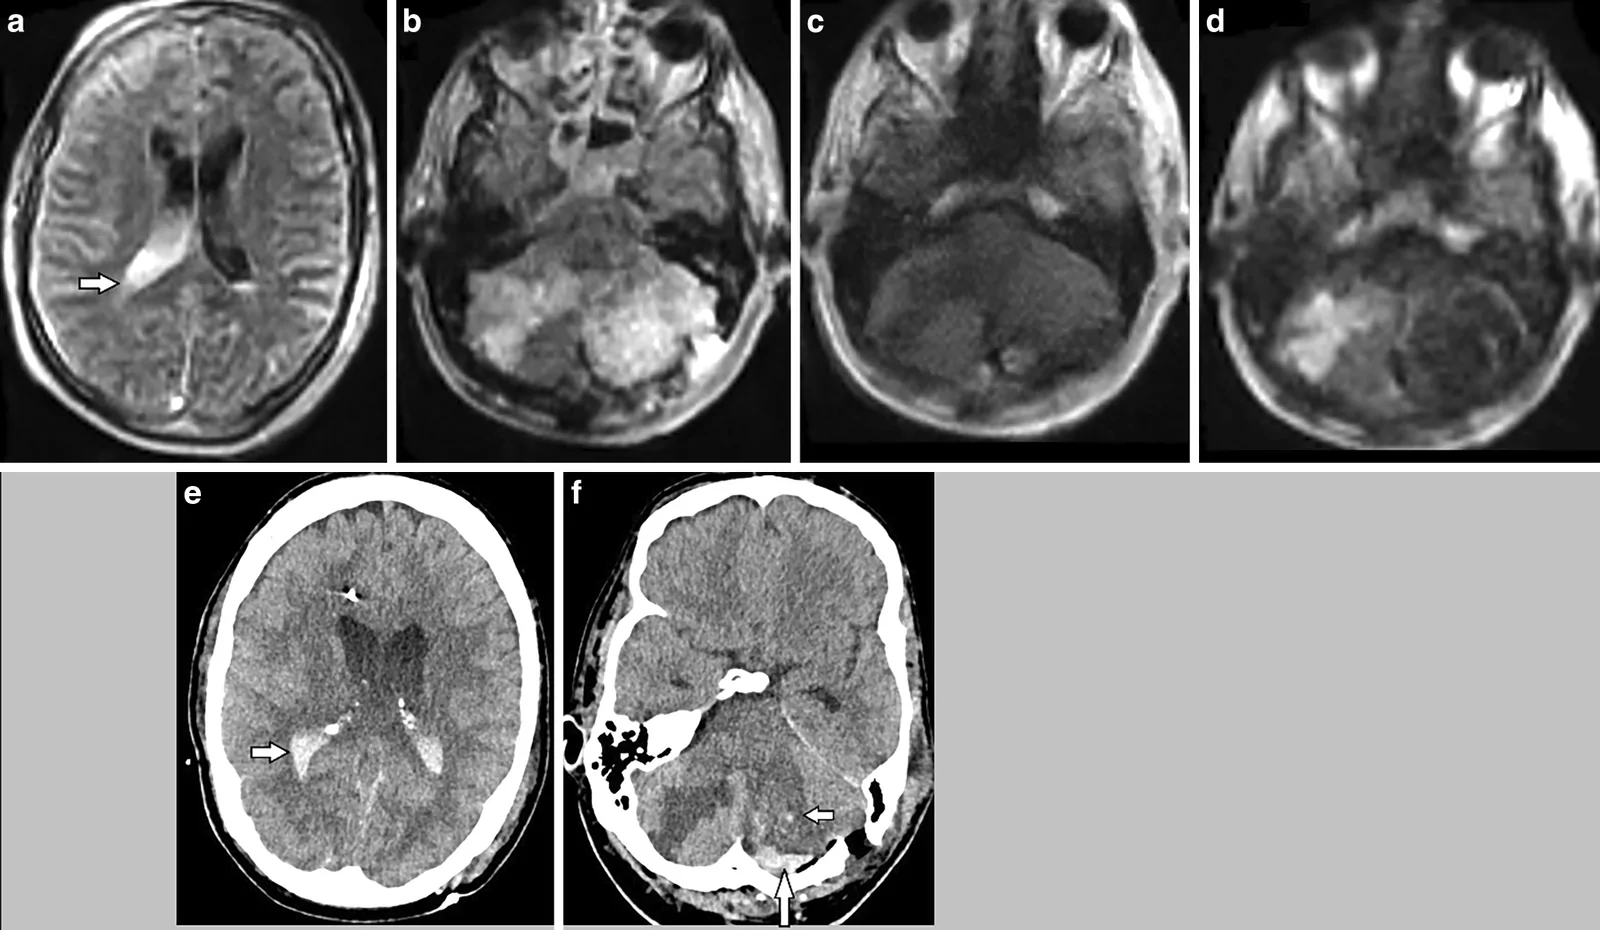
a 57-year-old patient with bilateral cerebellar infarctions with hemorrhagic transformation. ULF-pMRI showed massive intraventricular hemorrhage (HBC3b), predominantly in the right lateral ventricle (arrow in a FLAIR ULF-pMRI; e CT). Hemorrhagic components were clearly visible only on CT, which was obtained after surgical decompression for edema and impending herniation. Follow-up SWI MRI was performed one day later (infarct age: 4 days)
